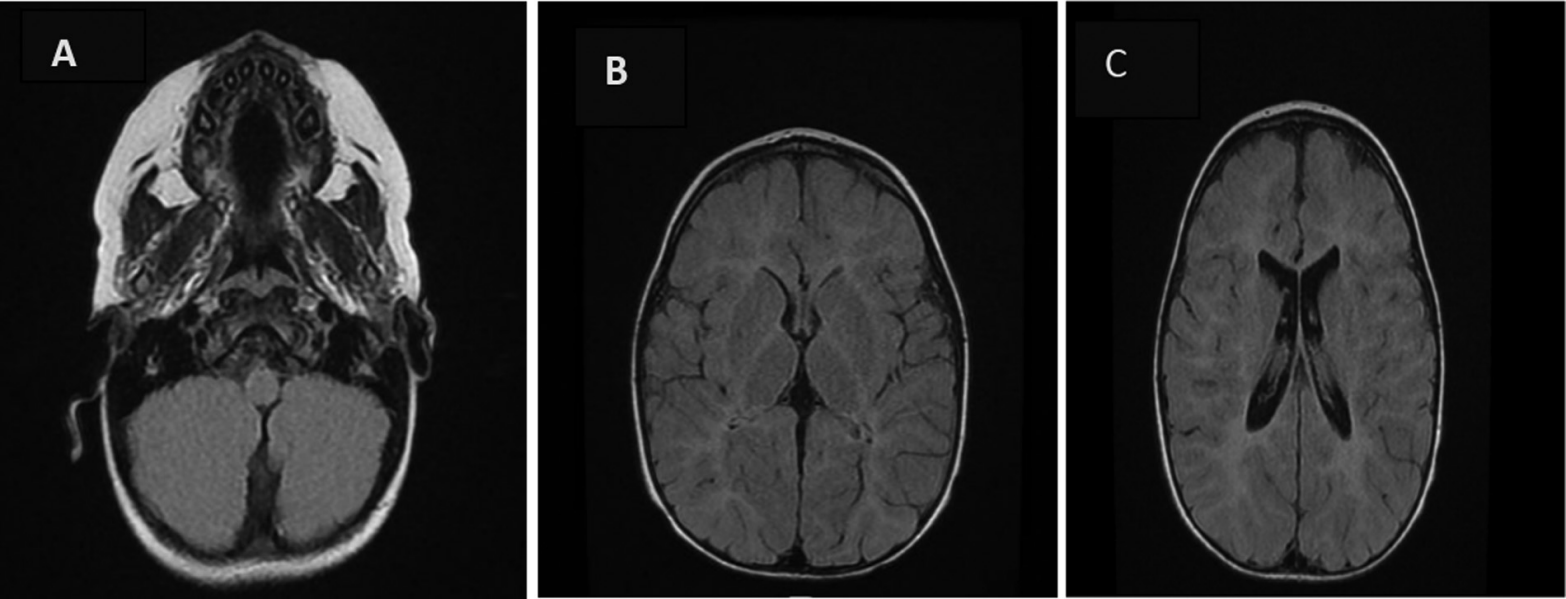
Fig. 1 (A–C) Brain magnetic resonance imaging (MRI) at the age of 4 years showed hypomyelination with a discrepancy between T1- and T2-weighted images and increased white matter signal intensity is seen on T2 and fluid-attenuated inversion recovery (FLAIR) images in the supra- and infratentorial brain involving the external and internal capsules as well as the corticospinal tract in the brainstem, indicating extensive demyelination.
